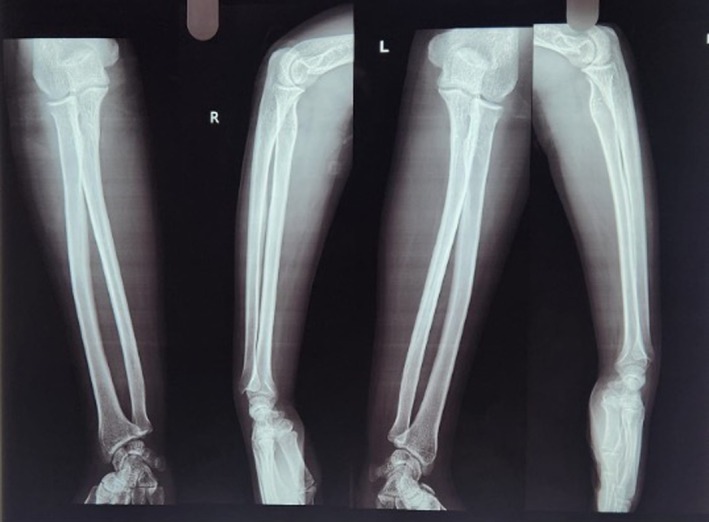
Case 2. Radiographs of the forearms and wrists showing absence of periosteal thickening, cortical irregularity, or other skeletal abnormalities.
